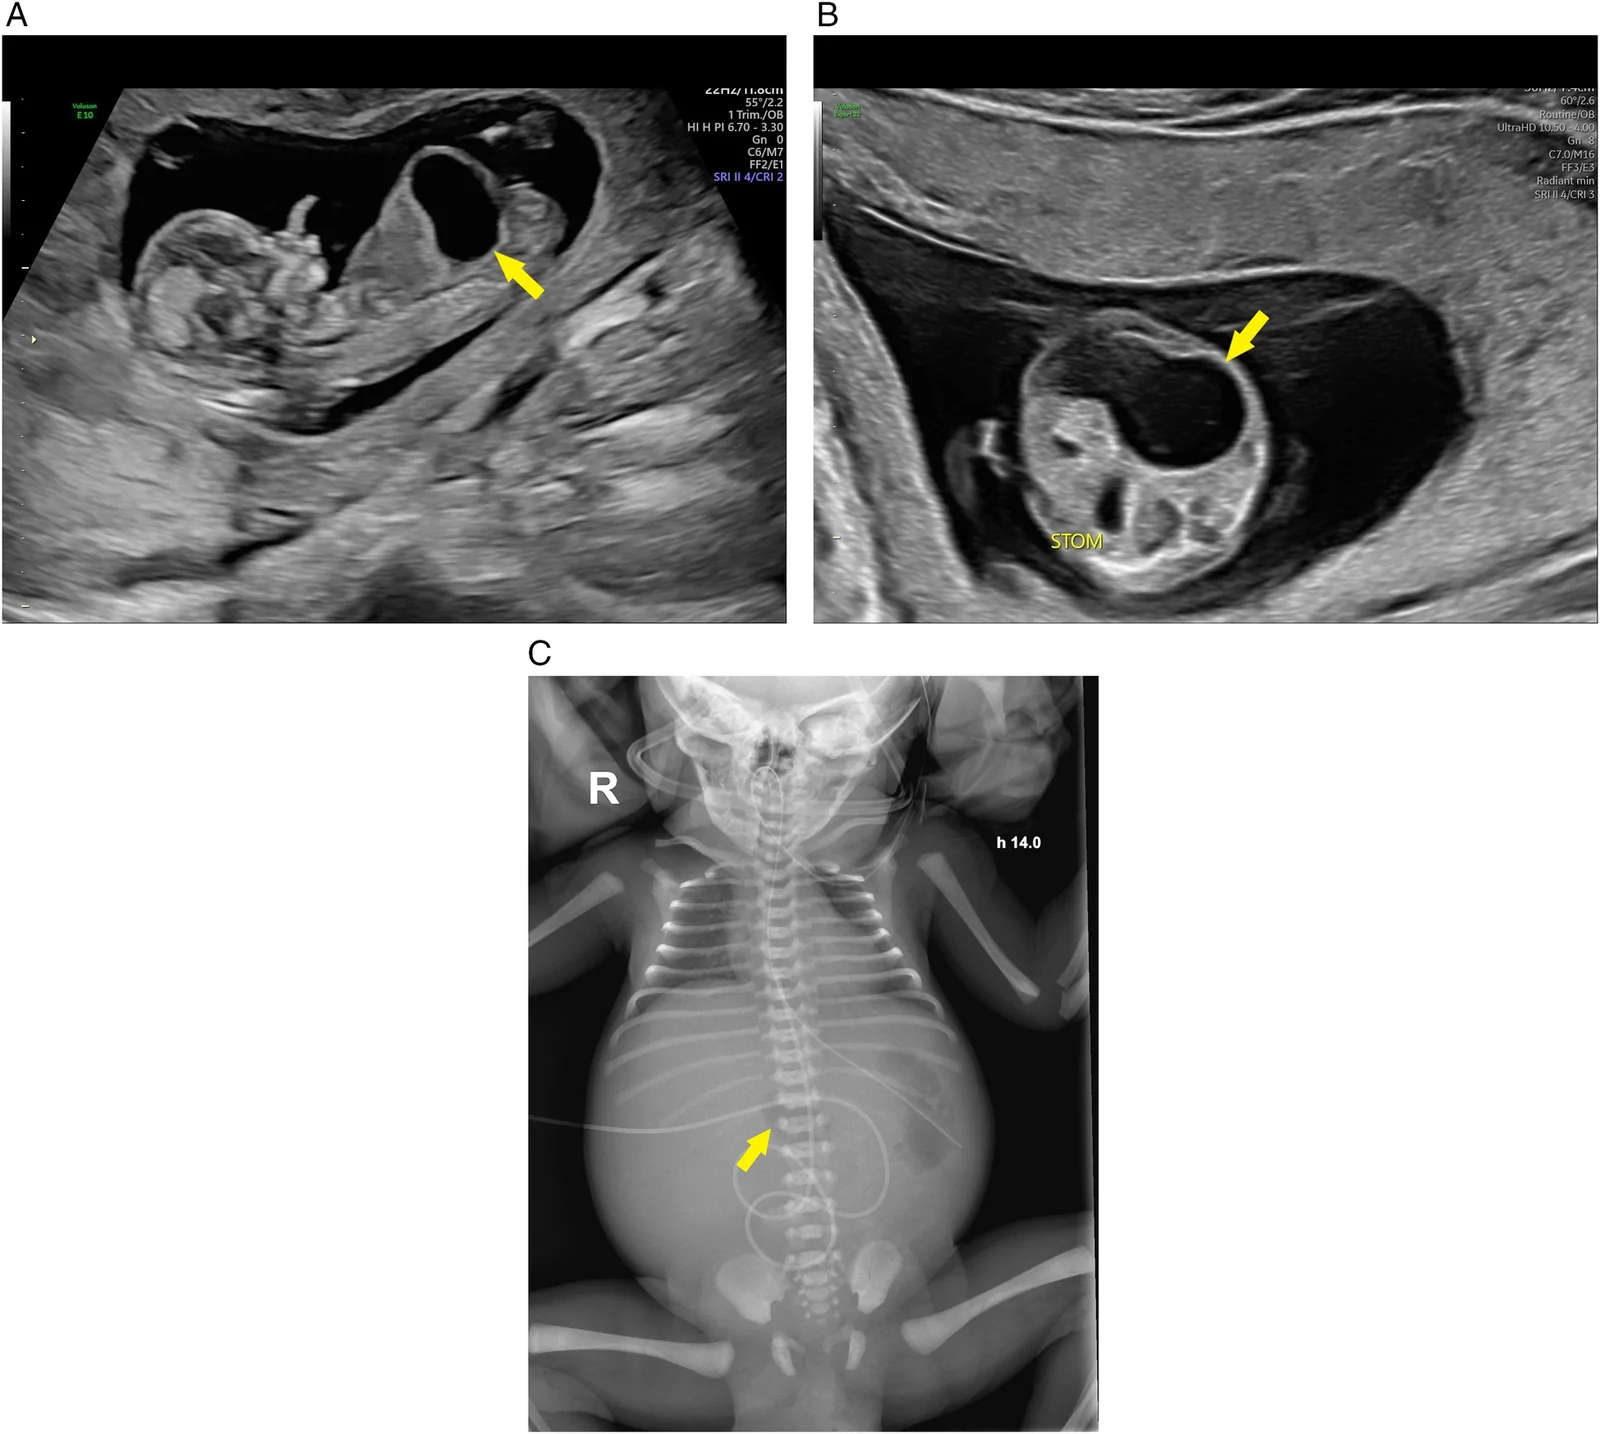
(A) Sagittal ultrasonography view of the fetus at 13 weeks of gestational age demonstrating a cystic lesion in the abdomen (*arrow*); (B) Axial ultrasonography view of the fetus confirming the presence of a cystic lesion (*arrow*) next to the stomach; (C) anteroposterior view of the x-ray at birth showing the stomach in the left upper quadrant and a single solitary bubble in the middle of the abdomen (*arrow*) highly suggestive of a closed duodenal loop syndrome.
